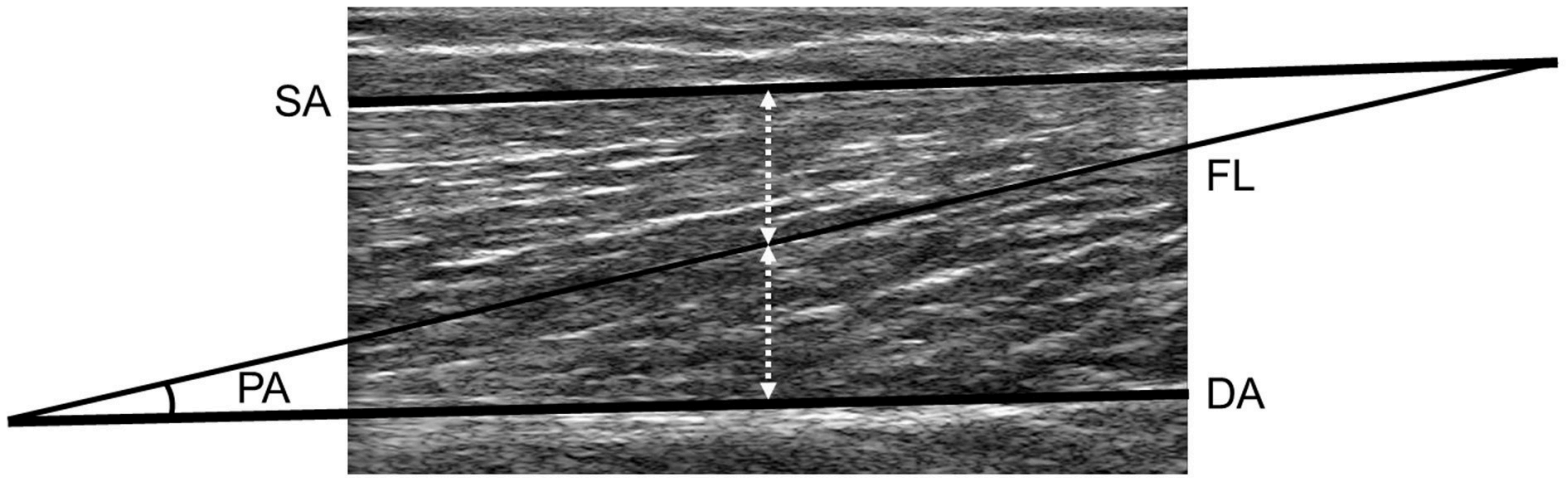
Ultrasound image of the long head of the biceps femoris. SA and DA lines represent the superficial and deep aponeurosis, respectively. FL corresponds to the fascicle length of the muscle fascicle, which joins SA and DA lines and crosses the midpoint between the two aponeurosis in the center of the image. PA represents the pennation angle, which was calculated as the angle between FL and DA.
